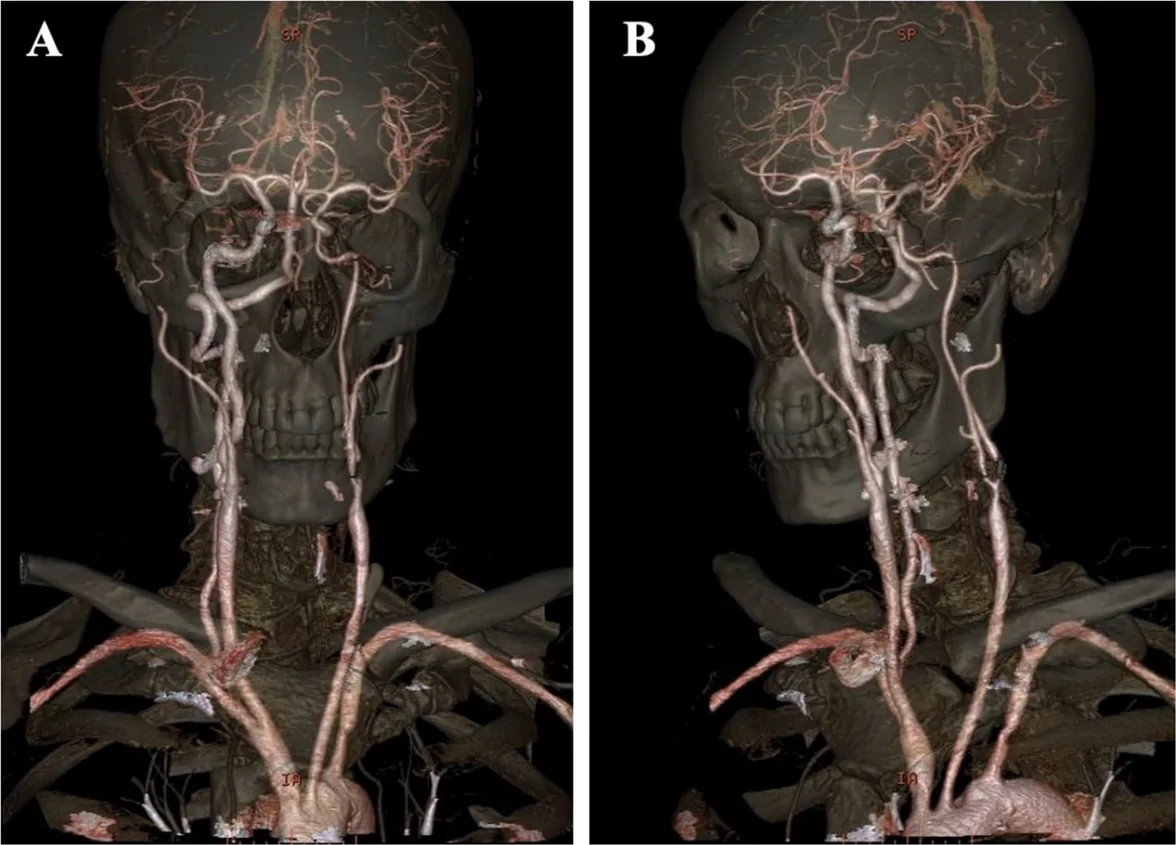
Original CT angiography images of the head and neck region. A. Frontal view, B. Sagittal view
